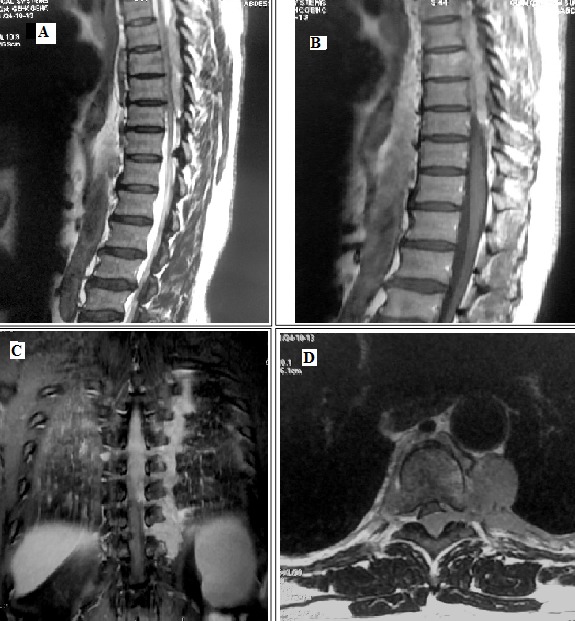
Spinal MRI in sagittal sequence in weighting T2 (A) and sagittal sequence (B); coronal (C) and axial (D) T1-weighted after gadolinium injection showed dorsal compression by an extensive posterior epidural tissue process from T6 to T8 in continuity with left pleural neoplastic thickening through the inter-vertebral homolateral foramens
